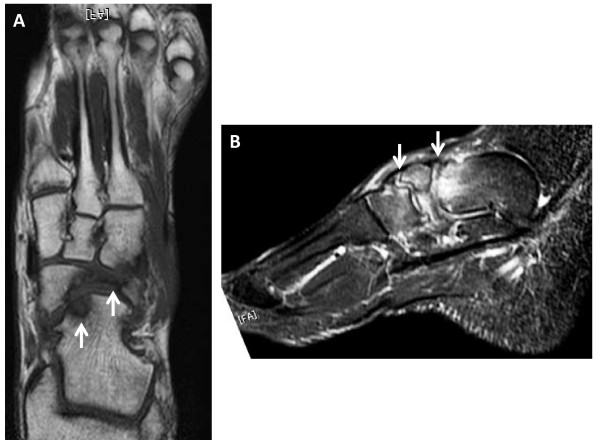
Preoperative magnetic resonance images. (A) Axial T1-weighted image showed avascular necrosis of the navicular bone and existence of a cystic lesion in the talar head. (B) Sagittal T2-weighted image showed slightly ragged talonavicular joint cartilage and incongruity of the talonavicular and navicular-cuneiforms joints.
